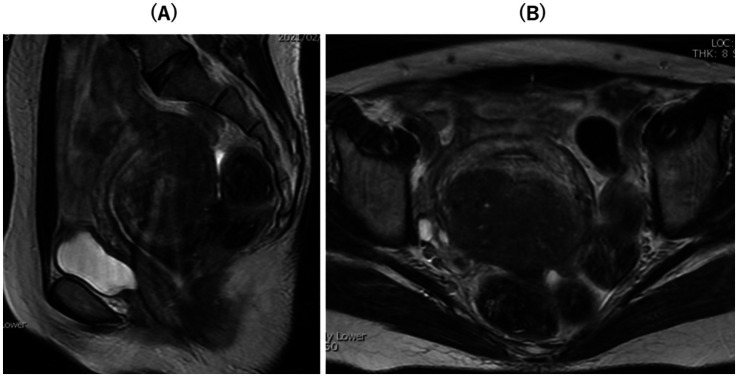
The preoperative T2-weighted magnetic resonance imaging (MRI). (A) Sagittal T2-weighted preoperative pelvic MRI showed diffuse adenomyosis with thickening of the junctional zone in the posterior wall and severe adhesion on the pouch of Douglas. (B) Axial T2-weighted postoperative pelvic MRI showed intramural solid adenomyosis.
